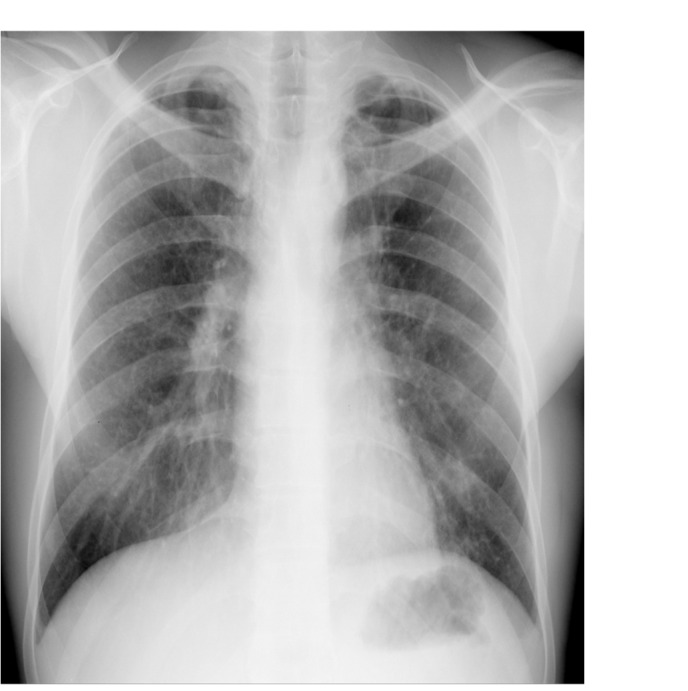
Chest X-ray after the right whole lung lavage
